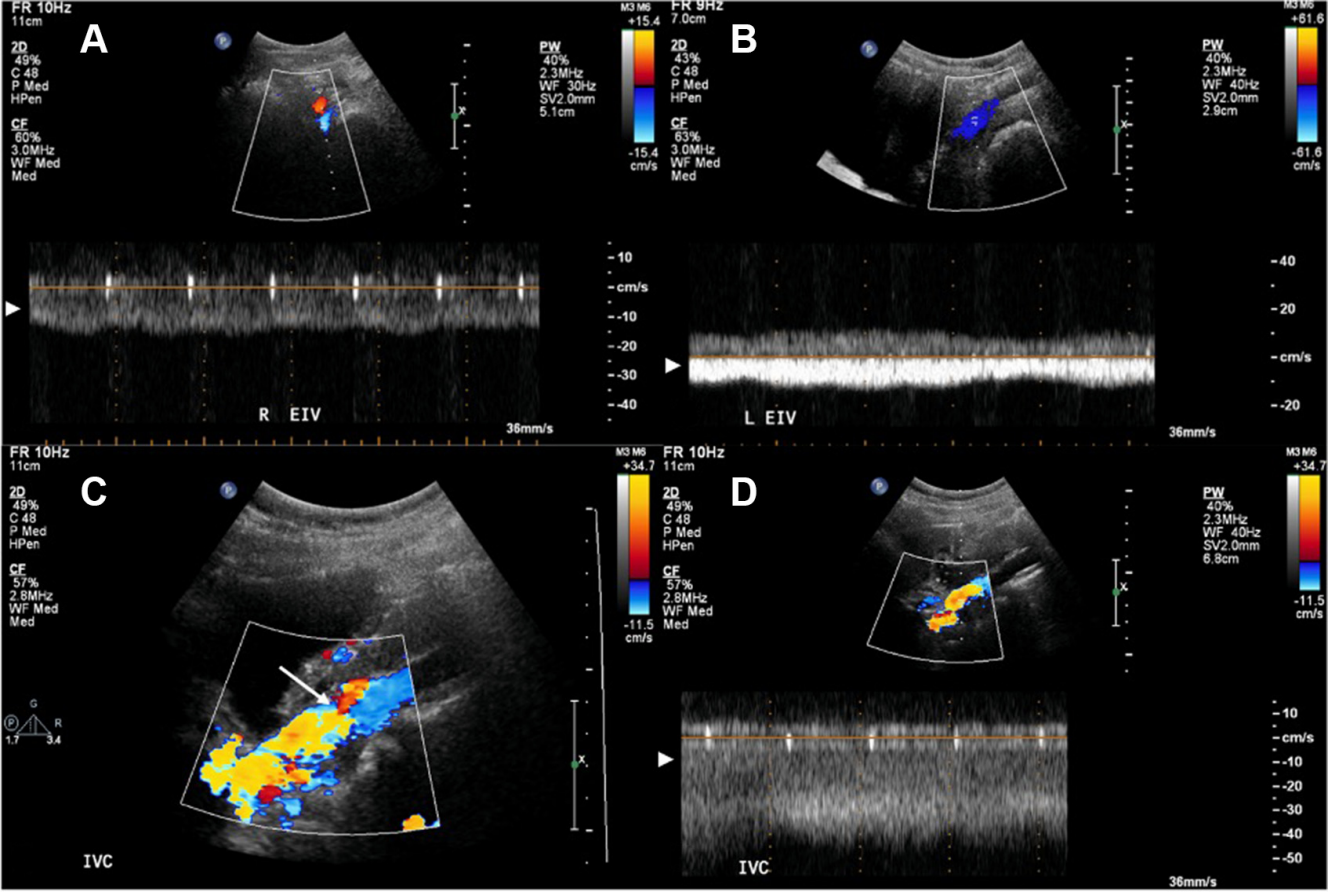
Bilateral External Iliac Vein and Inferior Vena Cava Doppler Ultrasound Doppler ultrasounds of the (A) right and (B) left external iliac veins shows continuous flow without respirophasicity, suggestive of a proximal occlusive lesion. Doppler ultrasounds of the inferior vena cava show high Doppler color-flow with (C) aliasing and an (D) abnormal continuous waveform pattern concerning for inferior vena cava obstruction.
